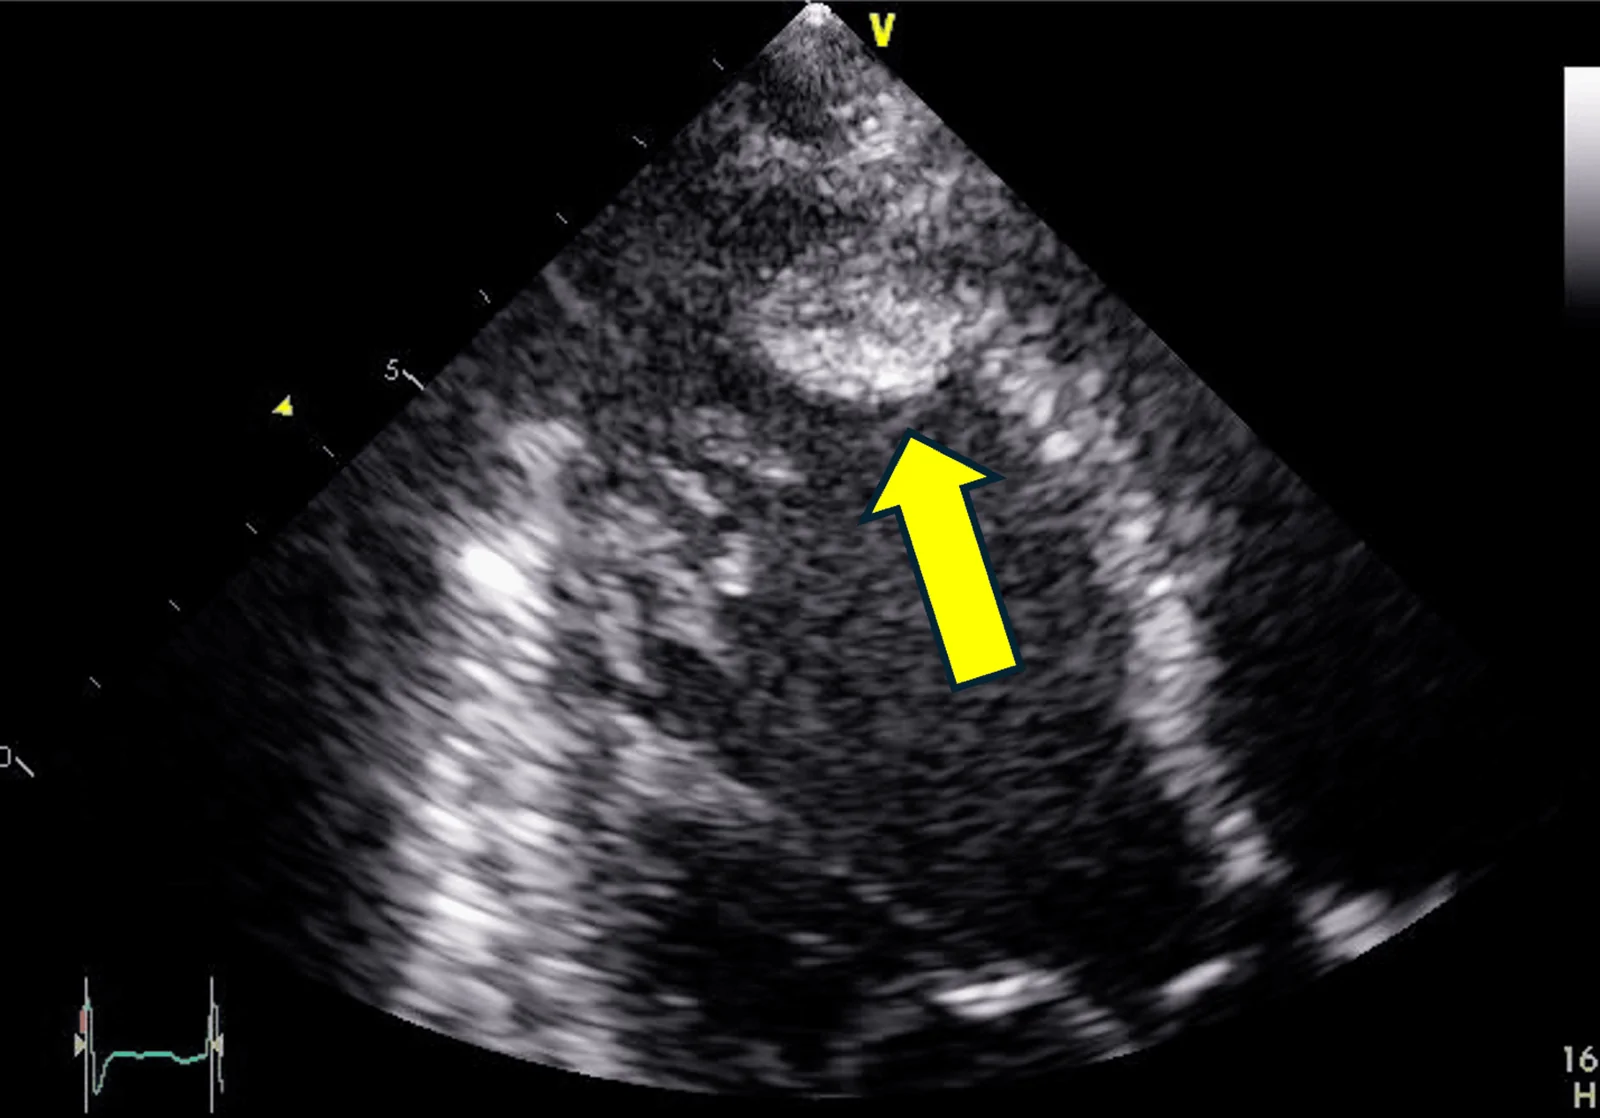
Transthoracic echocardiography on admission Apical four-chamber view demonstrating severe diffuse left ventricular systolic dysfunction with a left ventricular ejection fraction of 12%. A left ventricular thrombus attached to the apical wall is indicated by the arrow.
